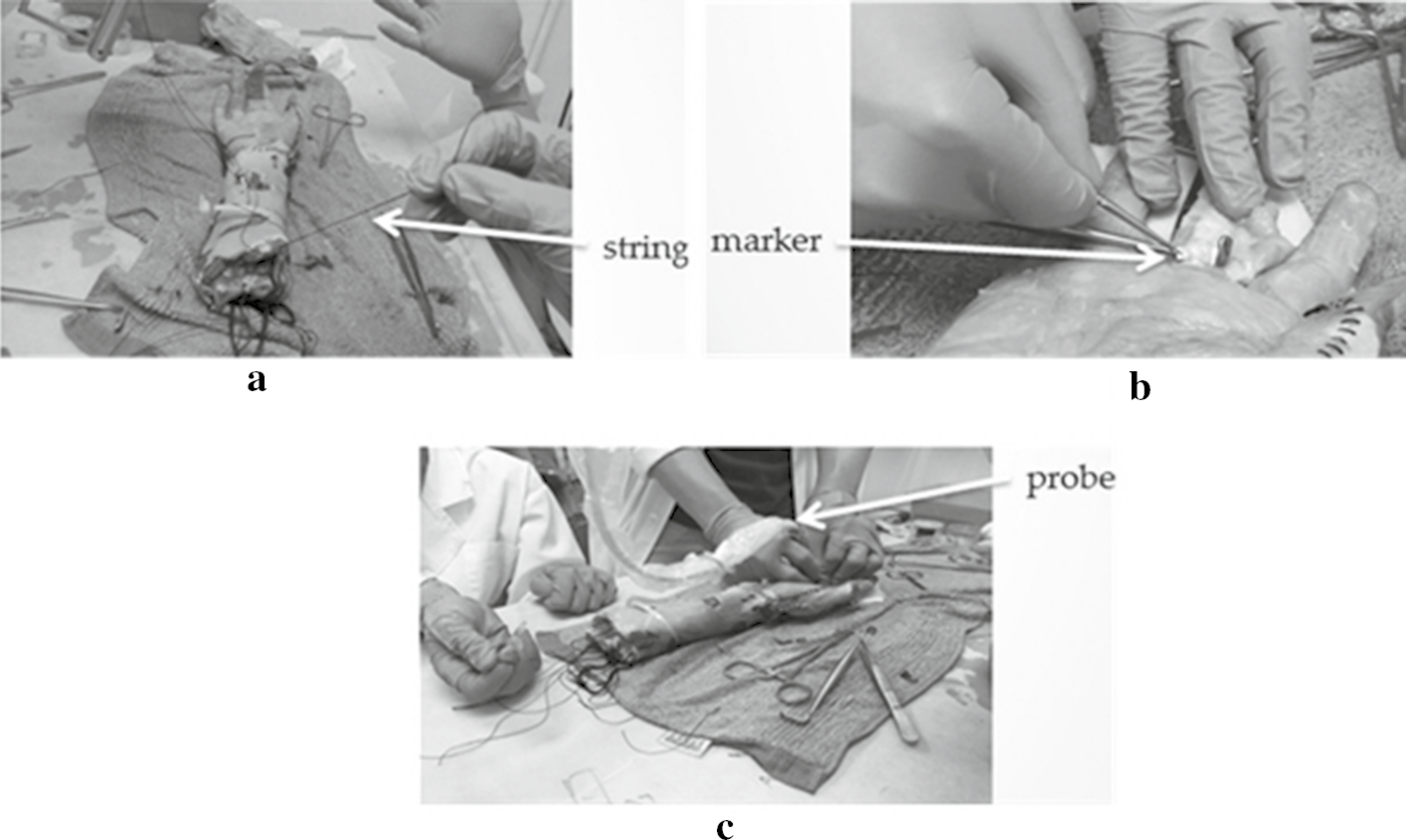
The cadaver’s hand used to acquire the ultrasound images. a The tendon tied with a string and passed through the entire forearm of the cadaver; b the marker inserted in the FDP tendon; c the acquisition probe placed above the A1 pulley position
